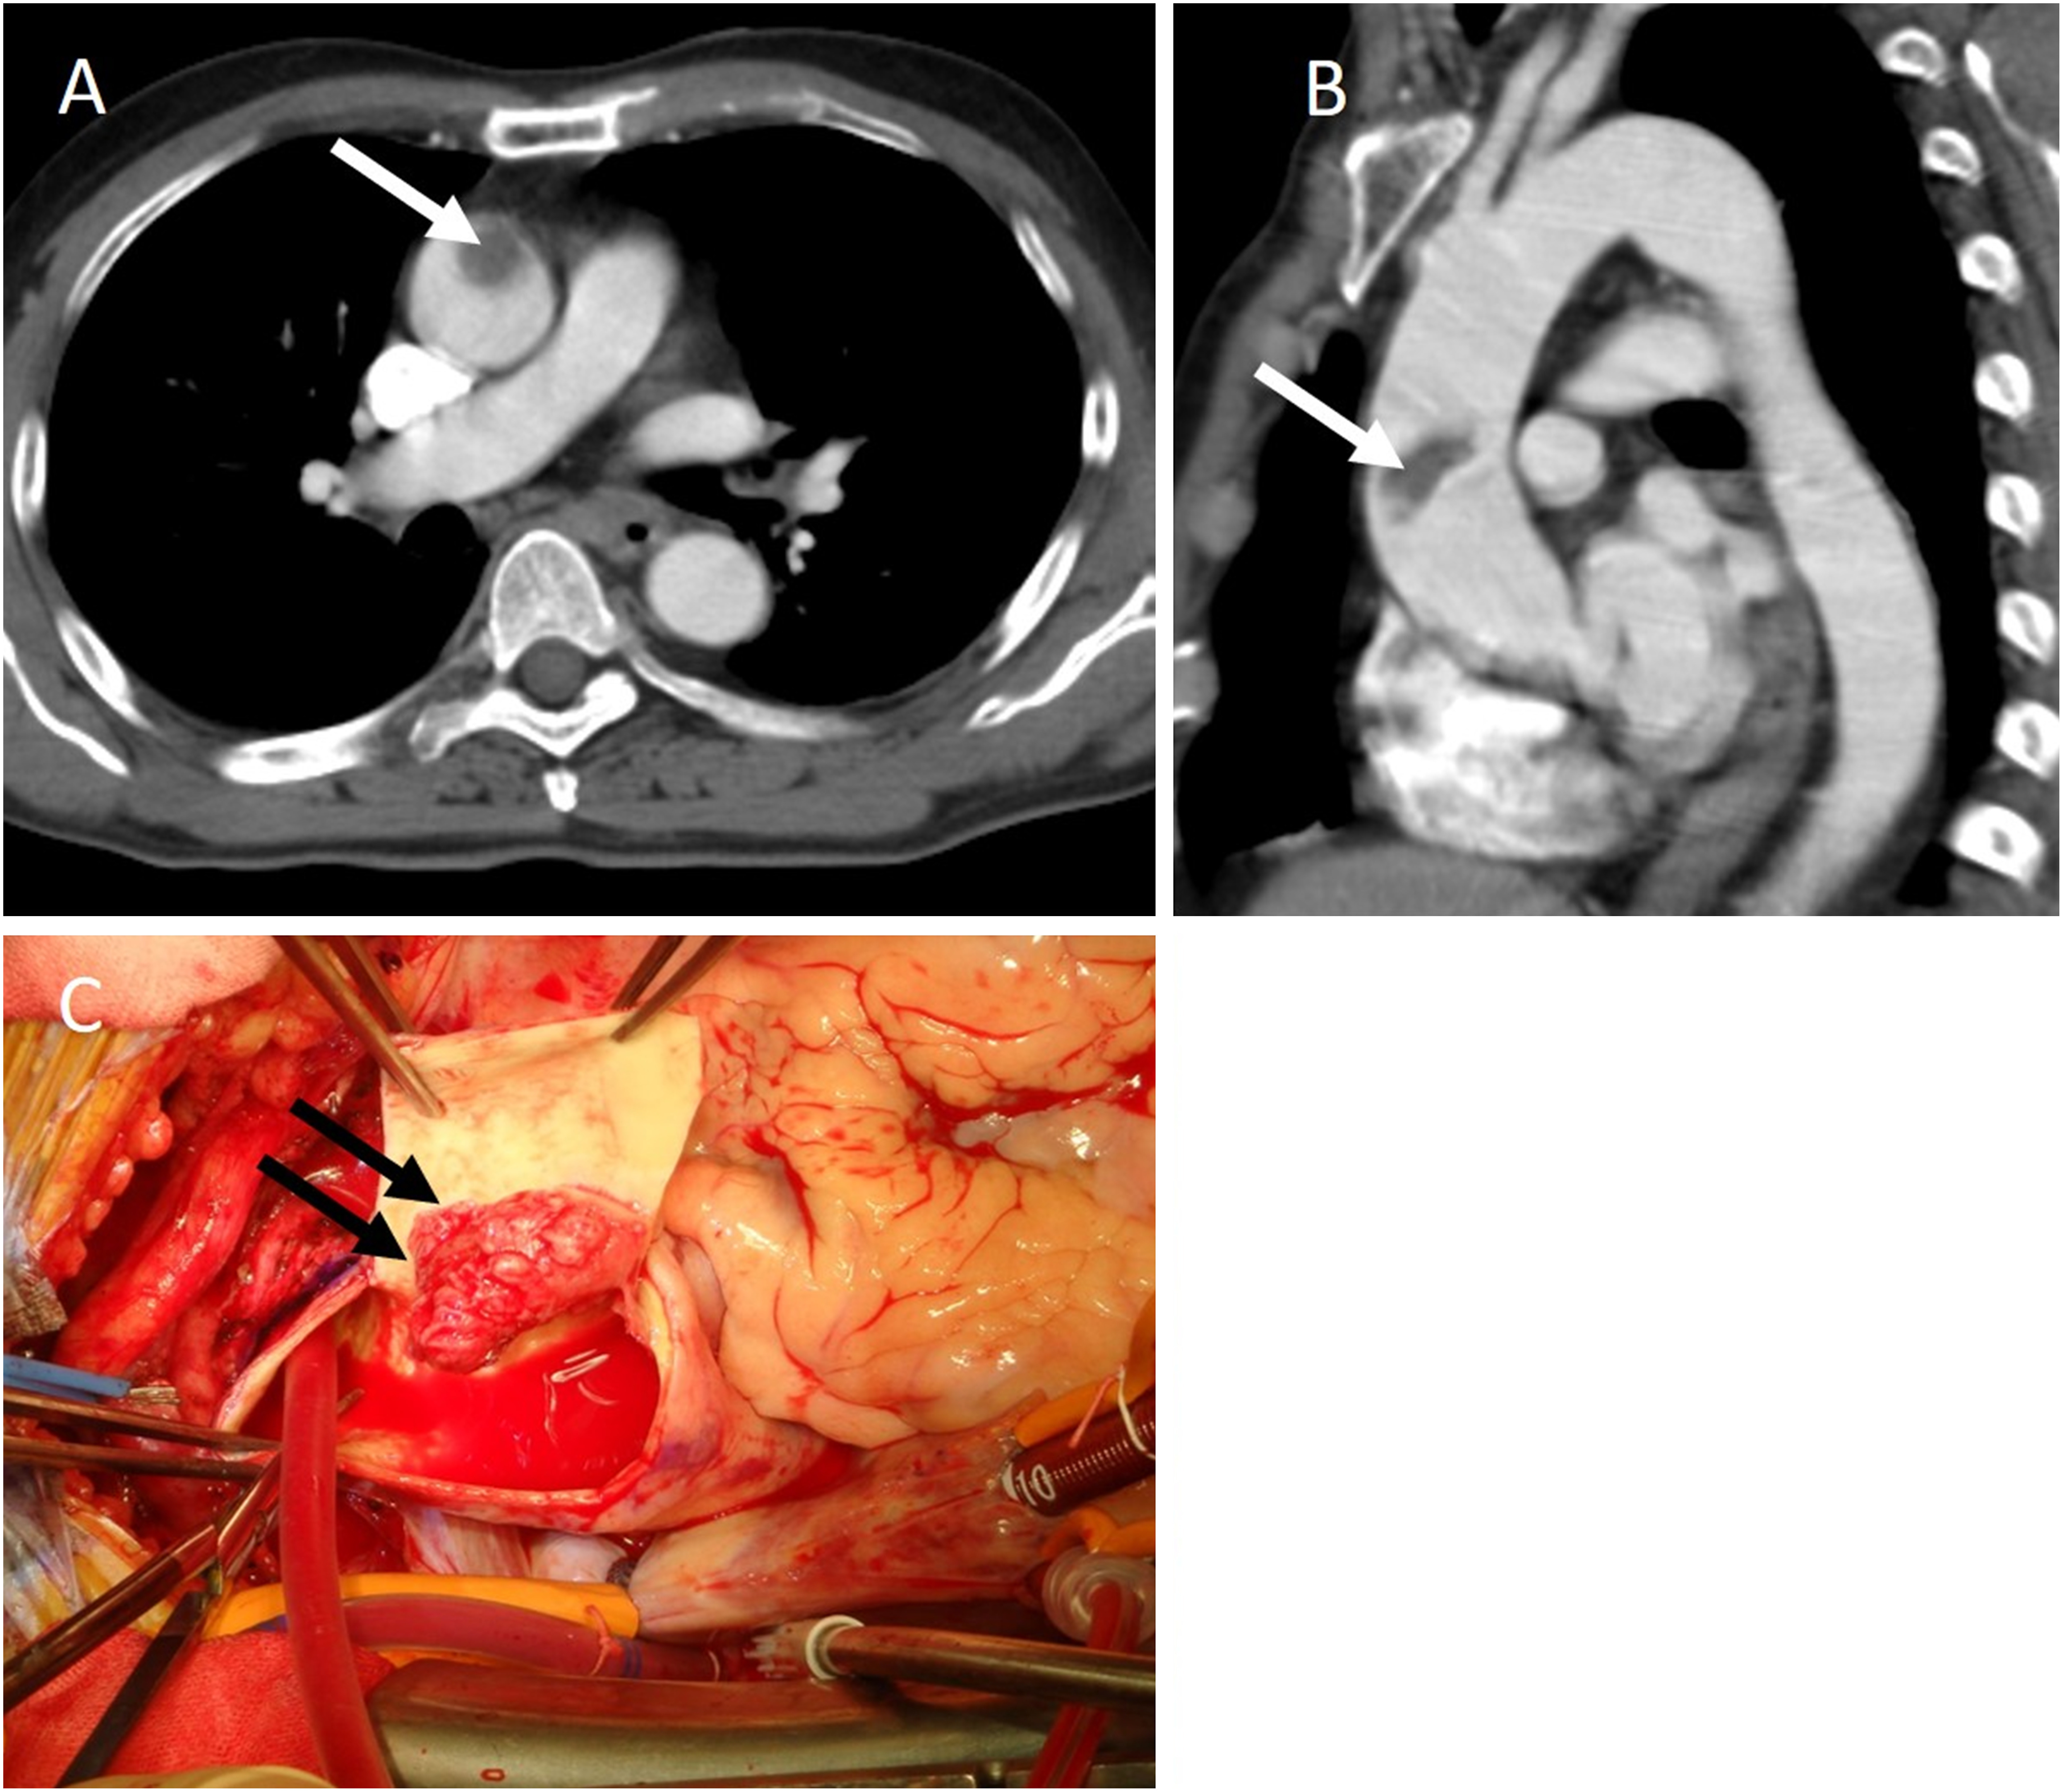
Fig. 1 (A, B) A contrast computed tomography showed thrombosis in ascending aorta (arrows). (C) In operative findings, the thrombus was size of 25×40 mm, fragile and high mobile (double arrows).
